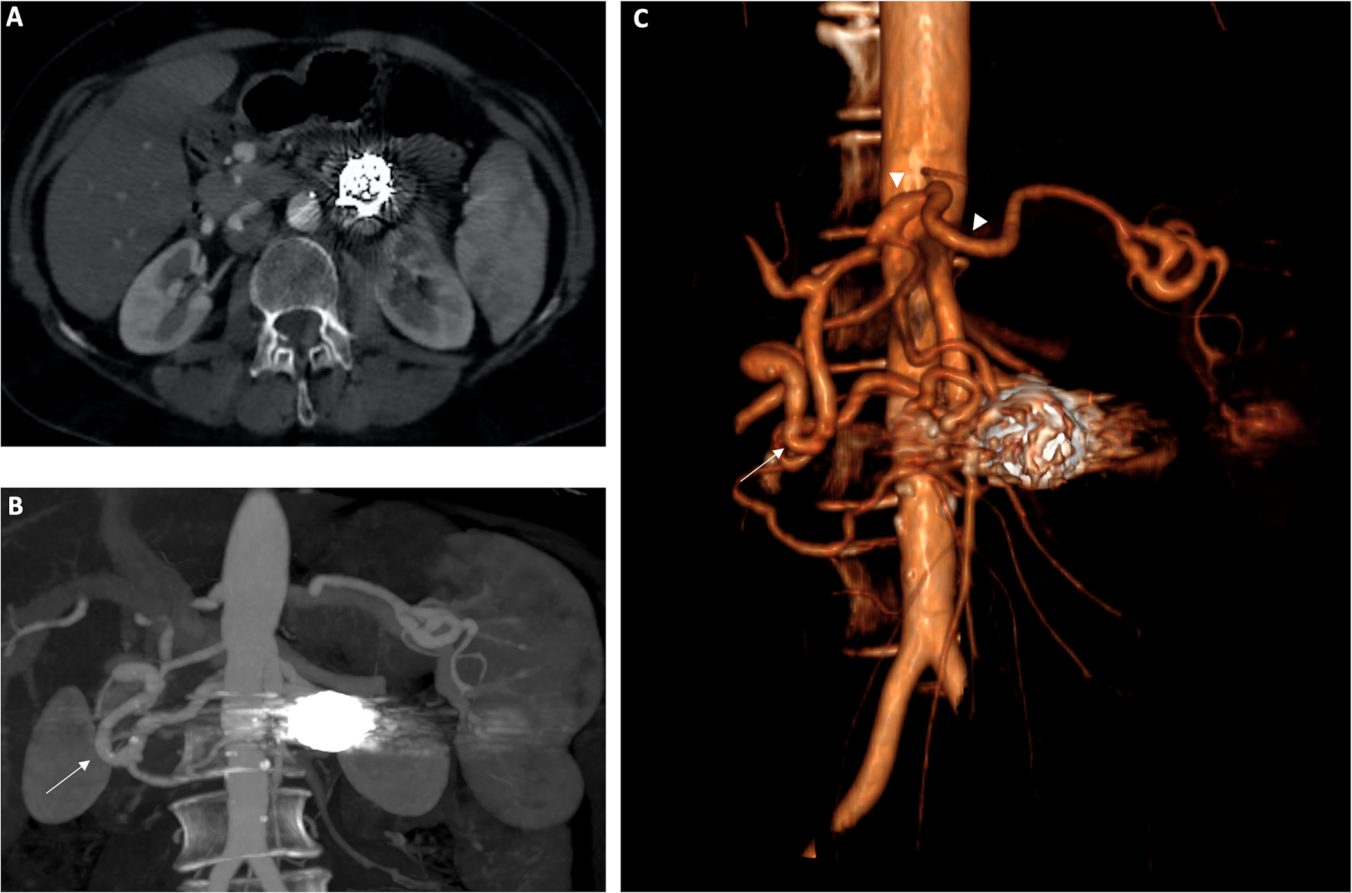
CT at 12 months showed complete exclusion of the aneurysmal sac from blood (a) flow with patency of inferior pancreatic duodenal artery and preserved vascularization of celiac branches (b, white arrow)
